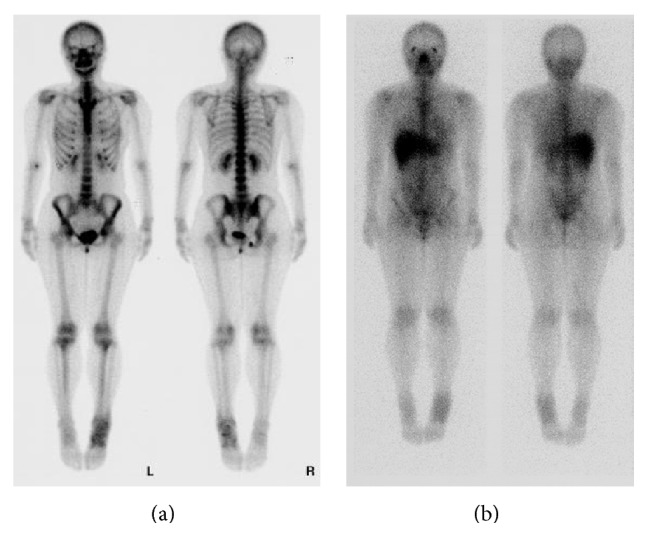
Bone (a) and Ga (b) scintigraphy show an increased uptake in the left ankle.
